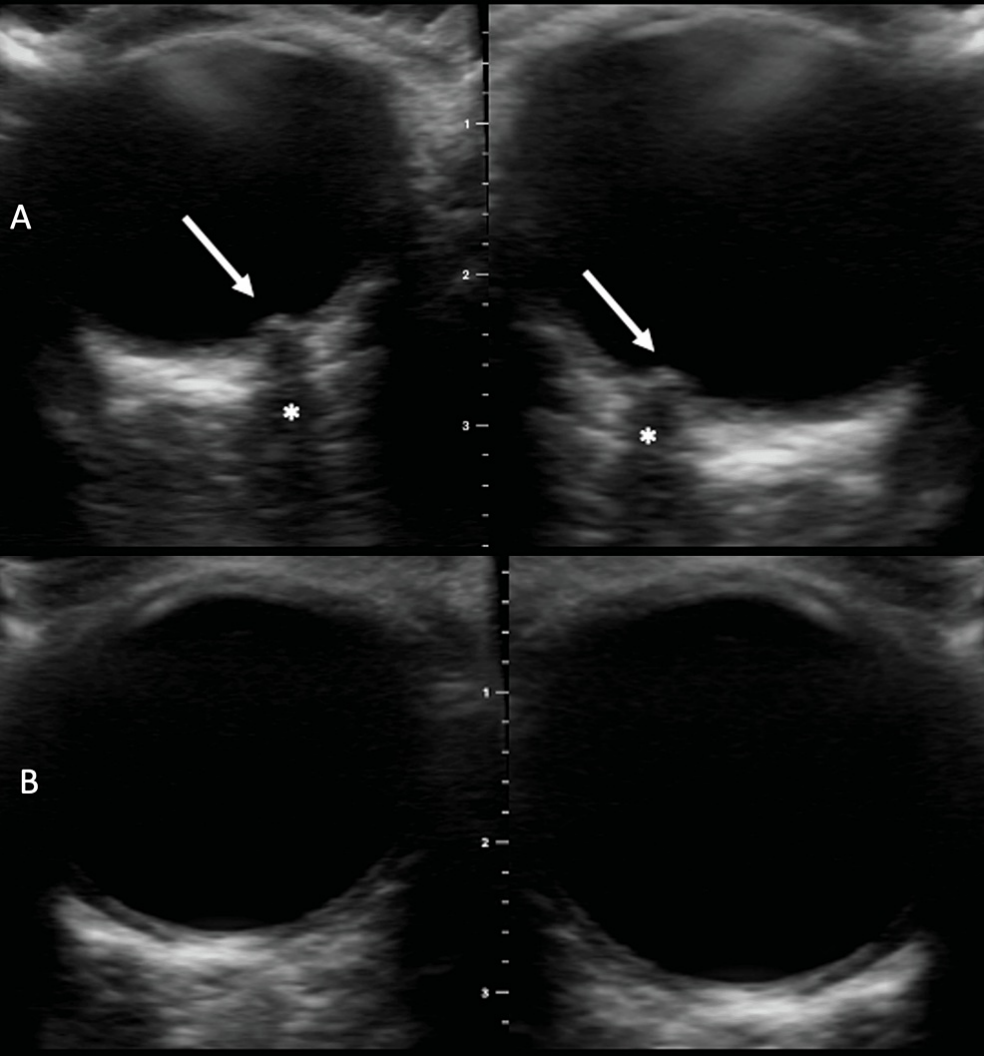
B-scan ultrasound image. 1A demonstrates marked optic disc elevation in the globes indicating raised intracranial pressure (arrow). Optic nerves indicated by *. 1B demonstrates resolution of optic disc elevation following the administration of mannitol for the management of increased intracranial pressure.
